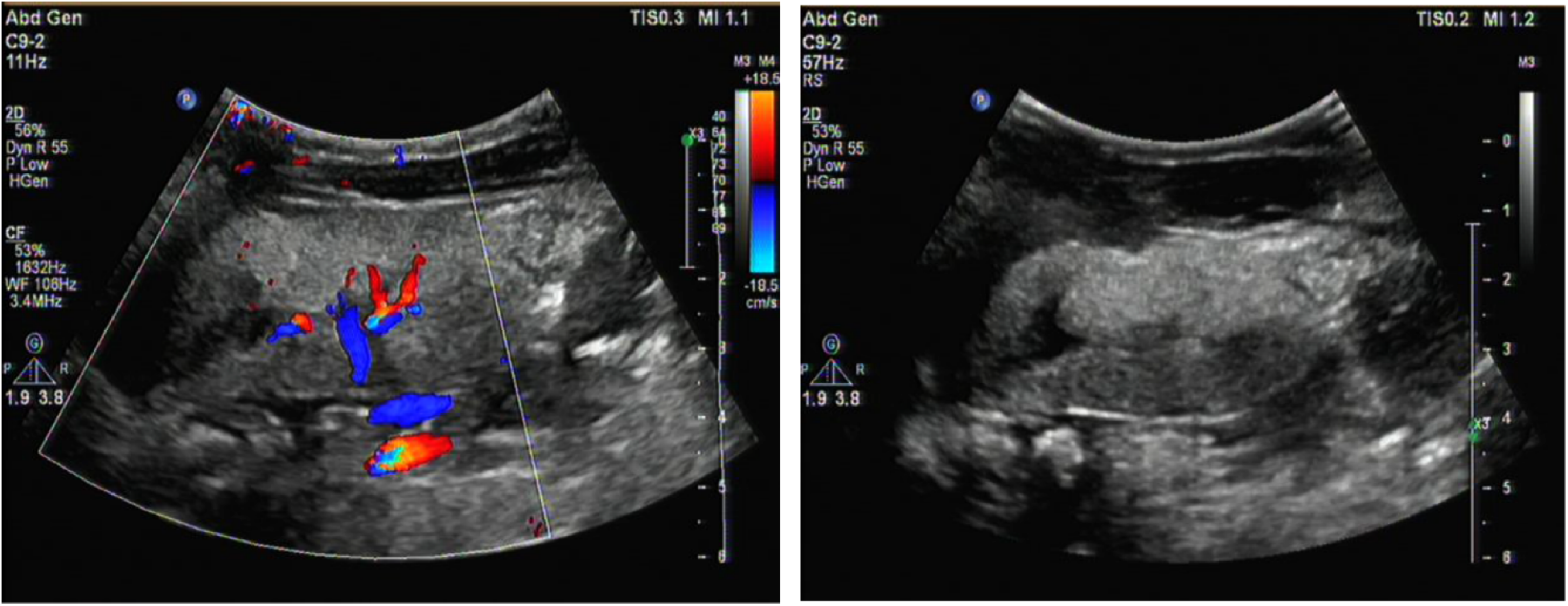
Renal ultrasound image. It revealed enhanced echoes of the parenchymaand, and unclear boundary between medulla and cortex of the bilateral kidneys as well as enlargement of both kidneys.
